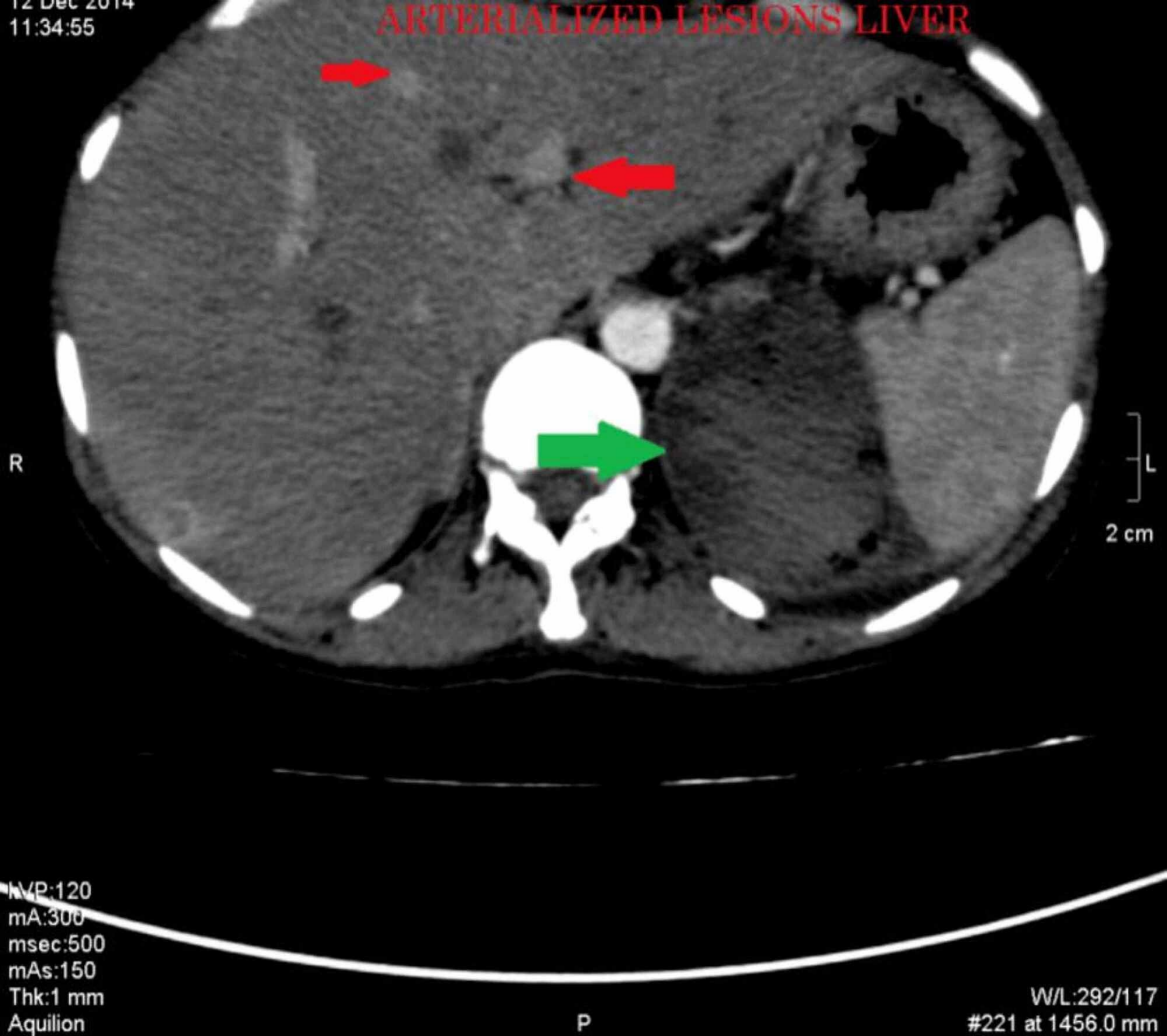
CECT abdomen axial section, arterial phase showing multiple arterialized lesions in the liver (red arrows) and a left subcapsular hematoma (green arrow) CECT: contrast-enhanced computed tomography
